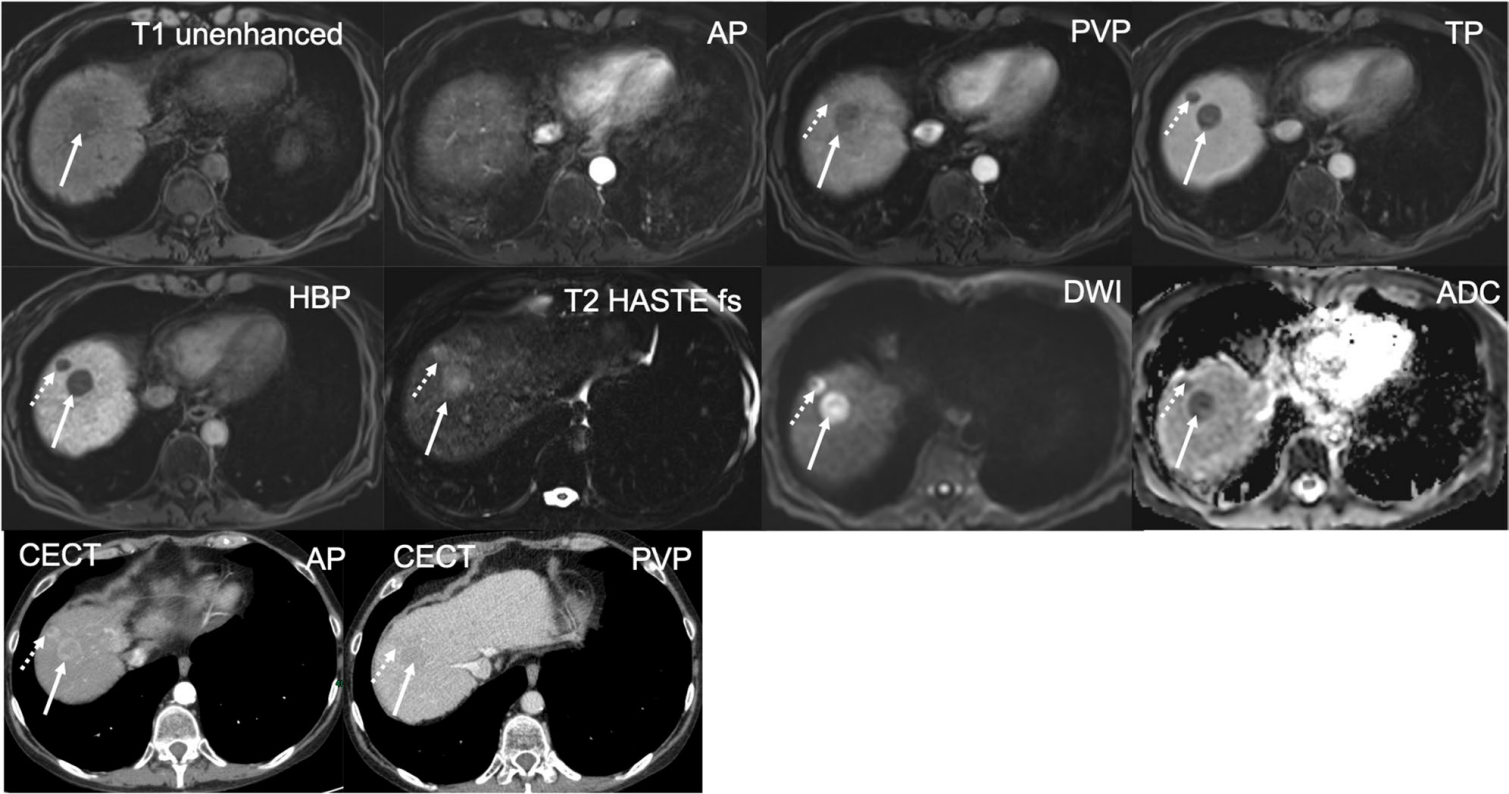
Severe TSM AP artifacts, score 4, suboptimal timing (too-early acquisition), poor lesion visibility, score 1: Blurring at boundaries between the liver and lung base indicating TSM. Neither lesion is assessable if compared with the HBP where they are now visible (score 1). This is a 79-year-old man with liver cirrhosis, Child–Pugh B and known HCC. On CECT, two HCC lesions with arterial phase hyperenhancement and PVP washout were seen in the liver dome. On MRI, the two HCC lesions are slightly T1 hypointense and T2 moderately hyperintense but barely seen in segment VIII of the liver on the AP due to too-early acquisition. In the PVP, both HCC lesions are hypointense and become better defined on the TP and HBP. Both lesions show diffusion restriction with high b values, b = 600
